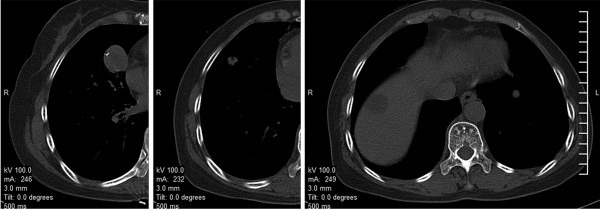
Computed tomography scan of Case 2. Multiple pulmonary sclerosing hemangioma in the medial and lateral segments of the middle lobe of the right lung and in the basal segment of the left lower lobe.
